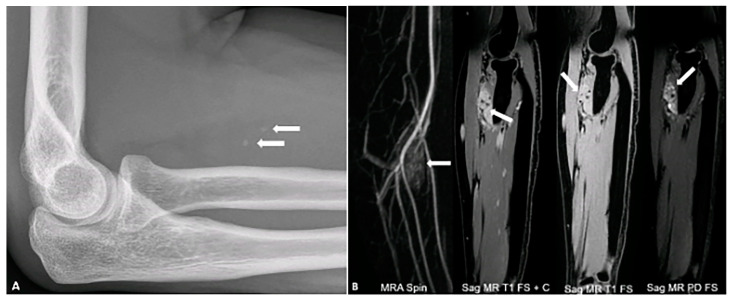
A 34-year-old female with right forearm pain. Lateral radiograph (A) reveals two foci of calcification (arrows) suggestive of phleboliths. Different MR sequences (B) show an enhancing mass (arrows) with hyperintense signal on the PD FS image, containing two internal foci of signal voids corresponding to phleboliths. The image was obtained from the University of Washington Medical Imaging database with the required permissions.
